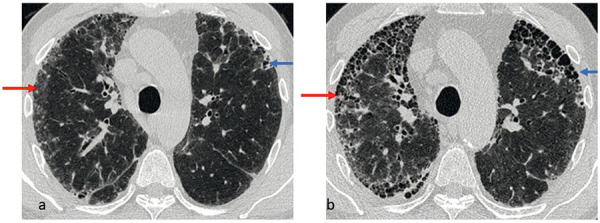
Noncontrast HRCT obtained at inspiration in fibrotic HP. Axial CT images at the level of the upper lobes. a: Initial findings; b: progression after 7 years. a: Irregular reticulations with traction bronchiolectasis subpleural, predominantly on the left (blue arrow). Subpleural on the right – next to subtle reticulation and traction bronchiolectasis – primarily with ground-glass opacity (red arrow). b: Progression of fibrosis in the previous areas of ground-glass opacities (red arrow). Retrospectively ground-glass opacities were the initial finding of fibrosis. Honeycombing in the subpleural areas of both lungs were new (see also the blue arrow in b, left upper lobe). Air trapping is not detectable in this case.
